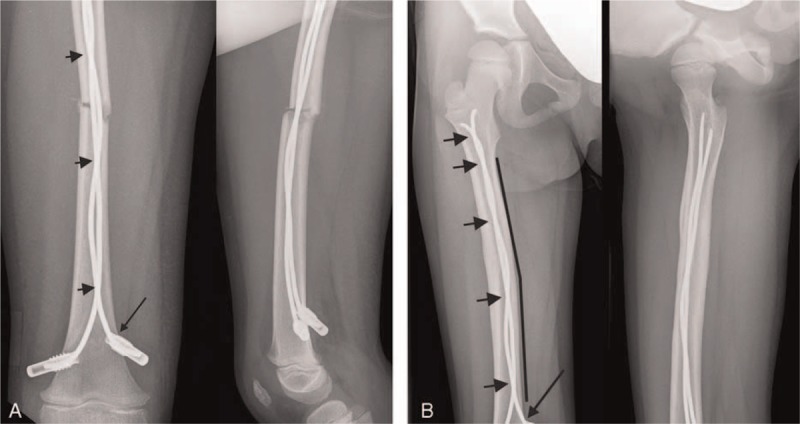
A 5-year-old girl suffered a transverse fracture of the femur during a fall down the stairs. Elastic intramedullary stabilization was performed on the day of injury. The radiograph obtained 10 days later showed a twisting of the nails (“corkscrew” phenomenon). Two EndCaps (long arrow) were inserted to increase the biomechanical stability of the osteosynthesis (A). Radiological consolidation of the fracture was documented after 5 months. There was a residual valgus angulation of 8°. The multiple crossings of the nails are indicated by arrows (B).
